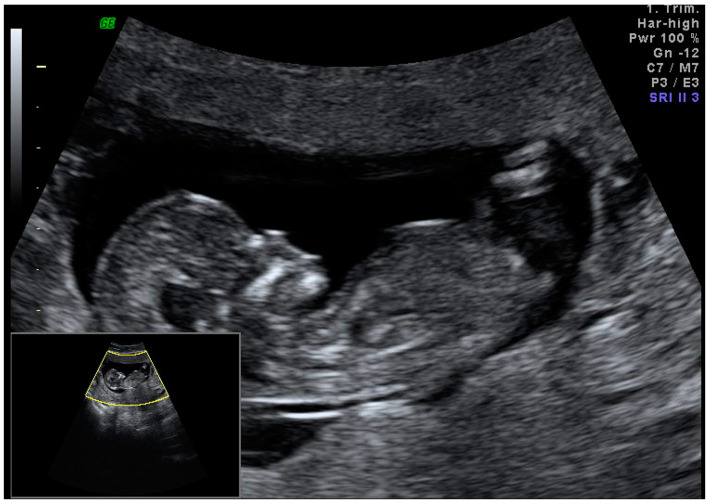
An ultrasound sagittal view of the fetus of 13 weeks and 2 days.
